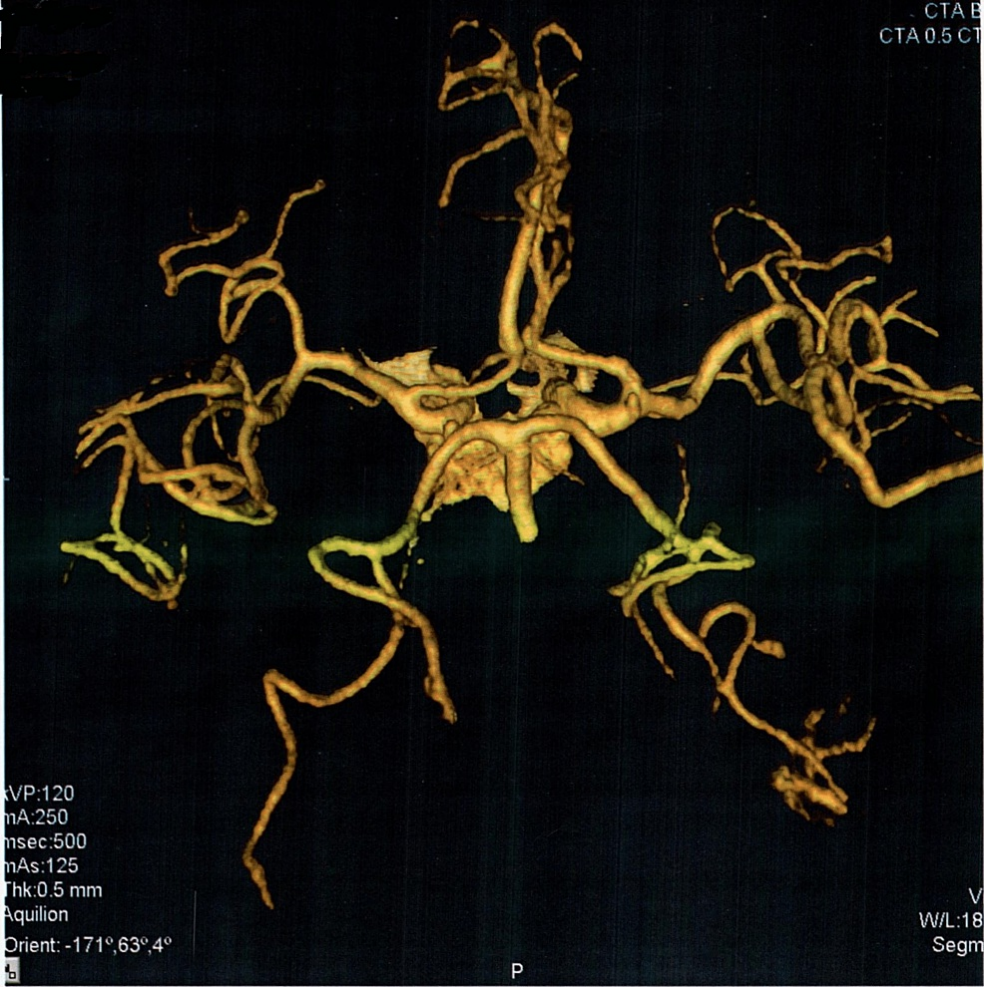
Computed tomography angiogram indicating cerebral blood flow
